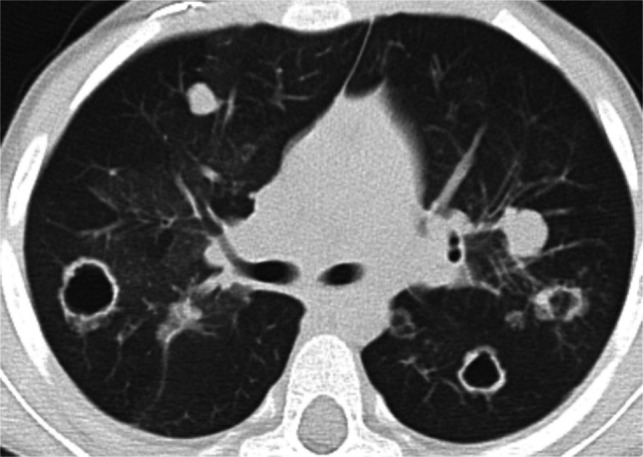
Recurrent respiratory papillomatosis in a 4-year-old male. Non-contrast axial chest CT shows bilateral solid and cavitary nodules
